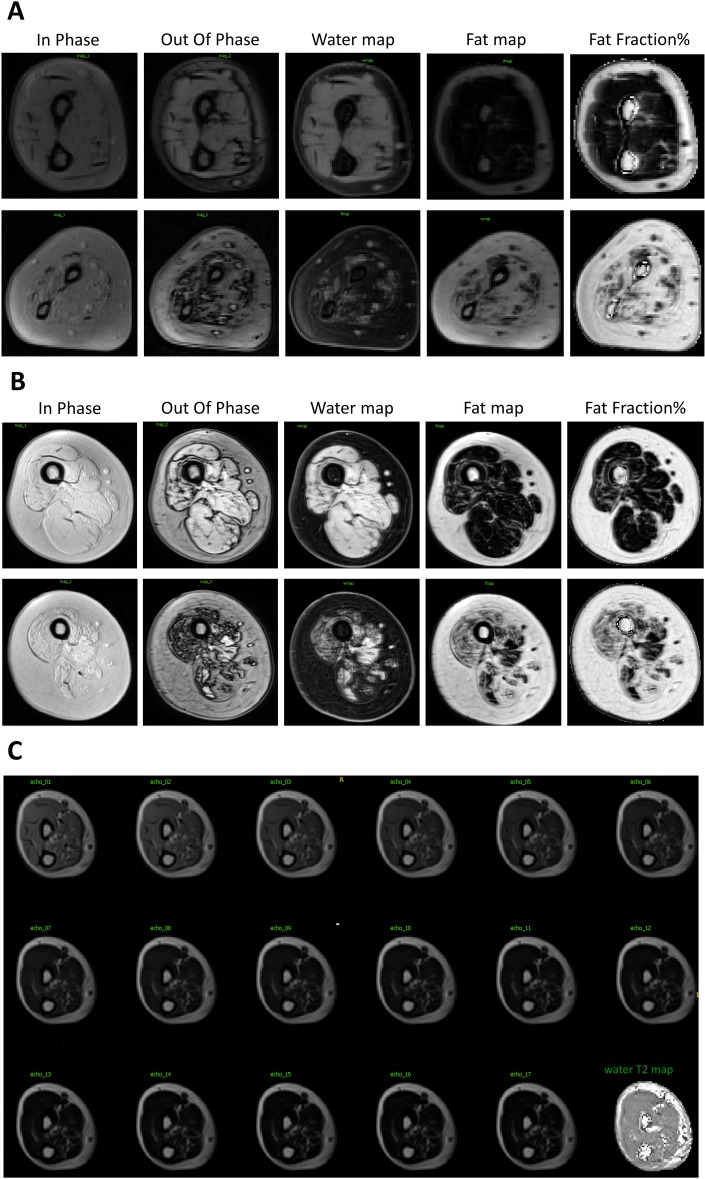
Example of MRI images of patients with SMA type 2 and 3. A. Example of Dixon images and water T2 map in mildly (upper panel; non-ambulant SMA type 3) and more severely (lower panel; non-sitter SMA type 2) infiltrated patient forearms; B. Example of Dixon images and water T2 map in mildly (upper panel) and more severely (lower panel) infiltrated ambulant patient thighs; C. Example of series of spin echo images at increasing TEs (10 ms steps) in the forearm of a patient and the water T2 map reconstructed from this series using the tri-exp fitting method.
